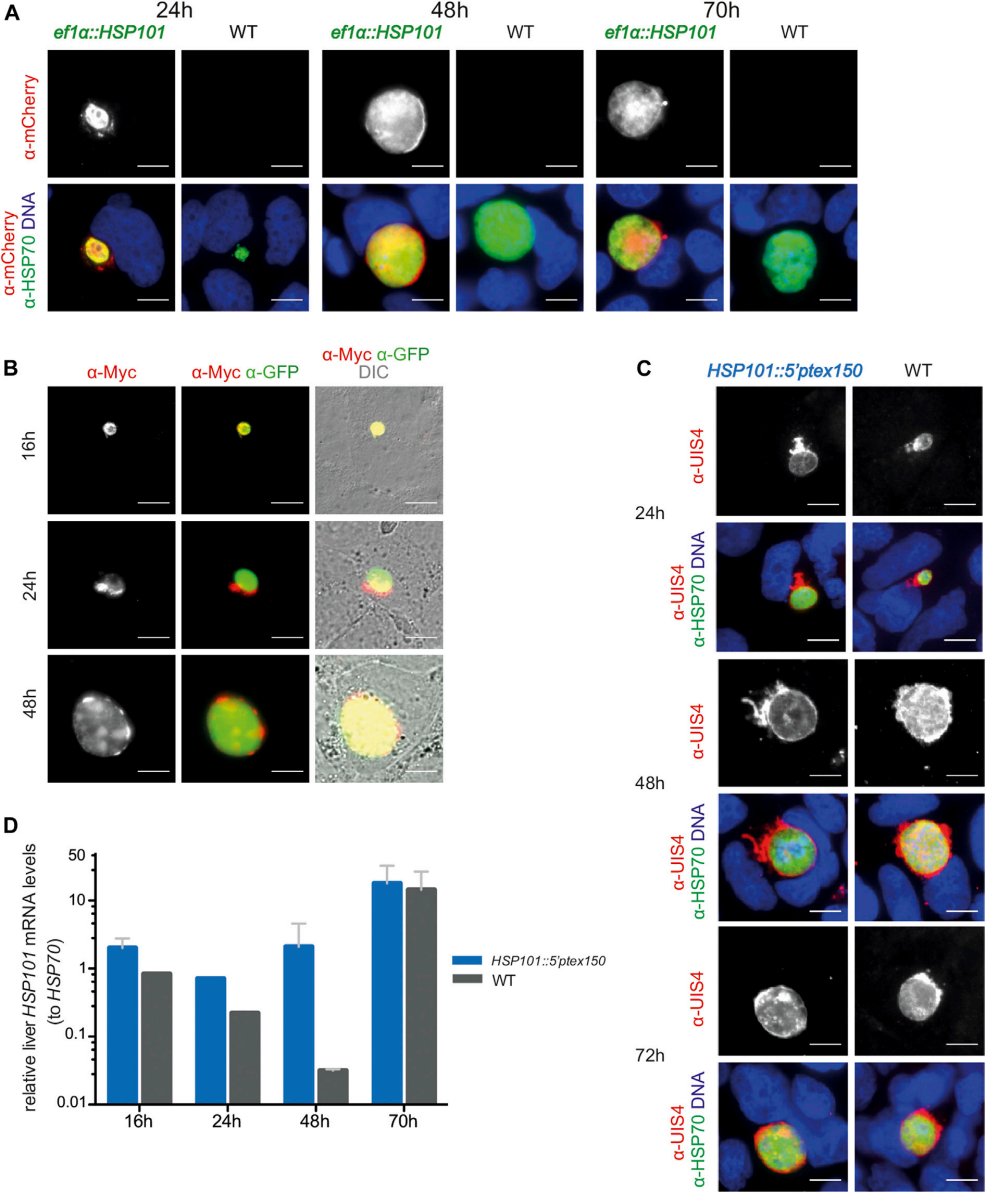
Ectopic expression of HSP101 in liver stages results in PV localization. (A) Localization of HSP101-mCherry in *ef1α:HSP101-mCherry*-infected hepatoma cells at 24, 48, and 72 h after infection. Shown are immunofluorescent images with *α*-mCherry antibody (top row) and merge images (bottom row) displaying the signals of *α*-mCherry (red), *α*-HSP70 (green), and Hoechst 33,342 (blue). Bars, 10 µm. (B) Localization of HSP101-myc in *ef1α:HSP101-myc*-infected hepatoma cells at 16, 24, 48 h after infection. Shown are immunofluorescent images with an *α*-myc antibody (left column), *α*-myc (red) and *α*-GFP (green) antibodies (center column), and merge images (right column) displaying the signals of *α*-myc (red), *α*-GFP (green), and DIC. Bars, 10 µm. (C) Liver stage development of *hsp101:5′ptex150* parasites at 24, 48, and 72 h after infection. Shown are immunofluorescent images with an *α*-UIS4 antibody (top), and merge images (bottom) displaying the signals of *α*-UIS4 (red), *α*-HSP70 (green), and Hoechst 33,342 (blue). Bars, 10 µm. (D) Steady state *HSP101* mRNA levels in *hsp101:5′ptex150* liver stages. Shown are relative mRNA levels determined qRT-PCR from total RNA of *hsp101:5′ptex150* or WT-infected hepatoma cells at indicated time points after infection. ∆∆CT values of *HSP101* mRNA values were normalized to *HSP70*. Depicted are mean values (±SD) (n = 2, except for 24h, n = 1).
